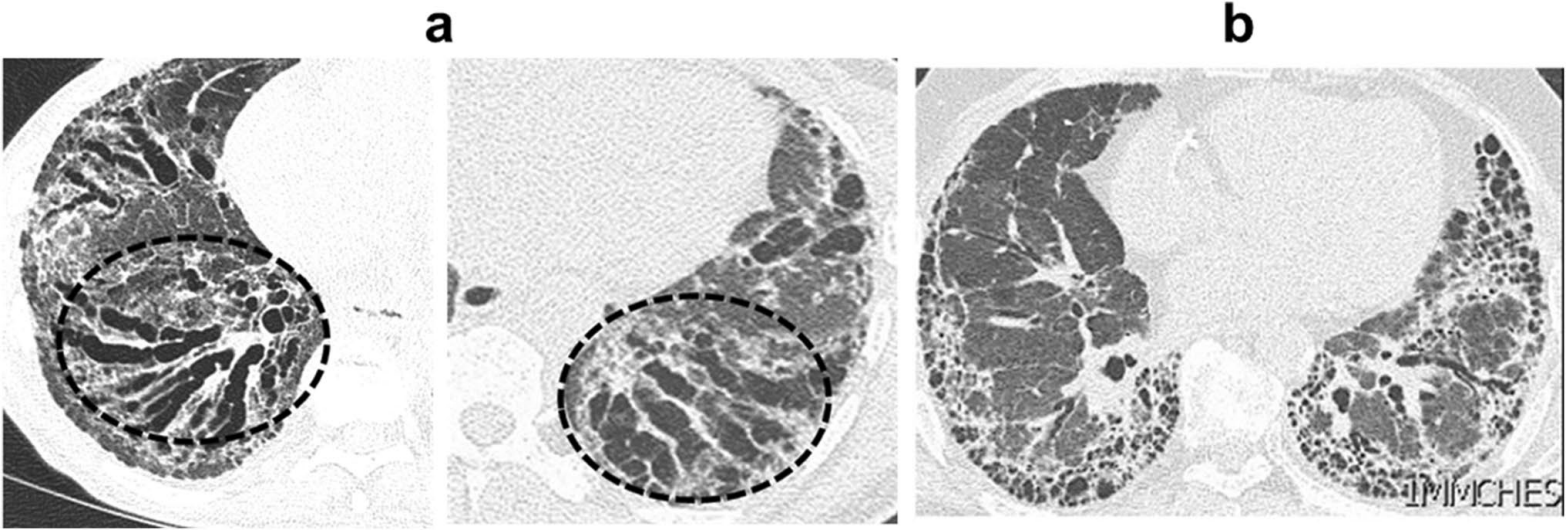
a Unenhanced axial chest CT images of two patients with SSc-ILD showing central traction bronchiectasis (highlighted within the black circles). b Chest HRCT scan of a patient with SSc-ILD and a peripheral usual interstitial pneumonia pattern of fibrosis. CT, computed tomography; HRCT, high-resolution computed tomography; SSc-ILD, systemic sclerosis-associated interstitial lung disease
